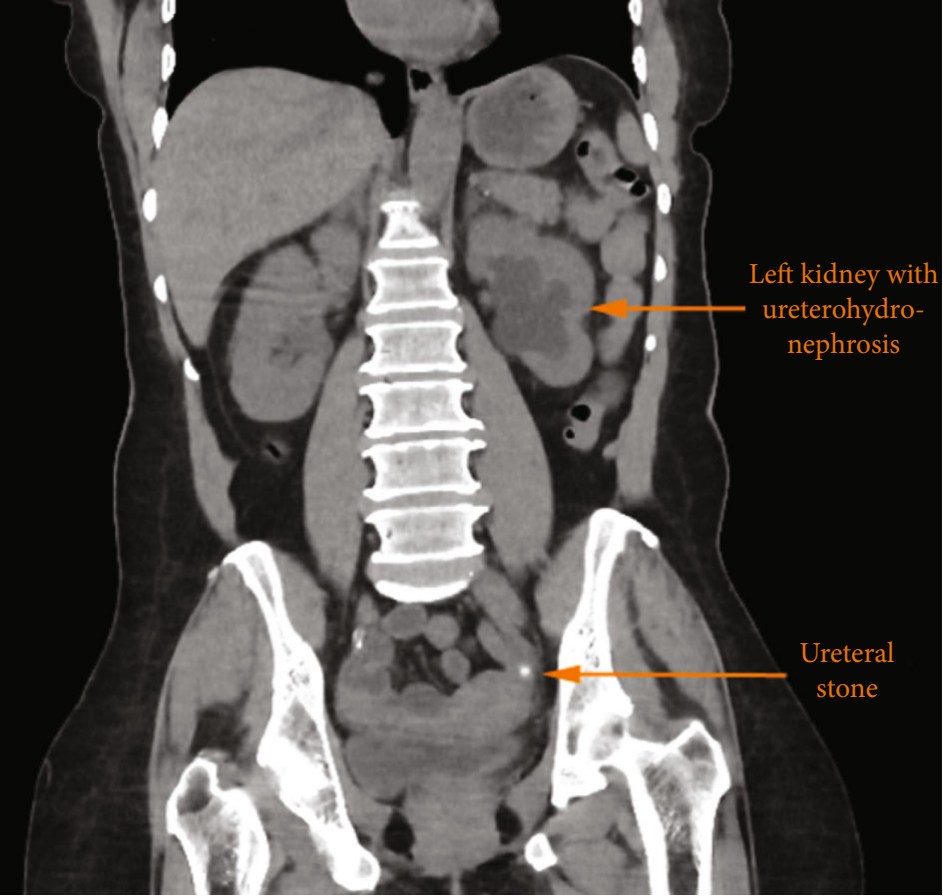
Abdominal computed tomography showing a hypotrophic left kidney with ureterohydronephrosis and a ureteral stone.
